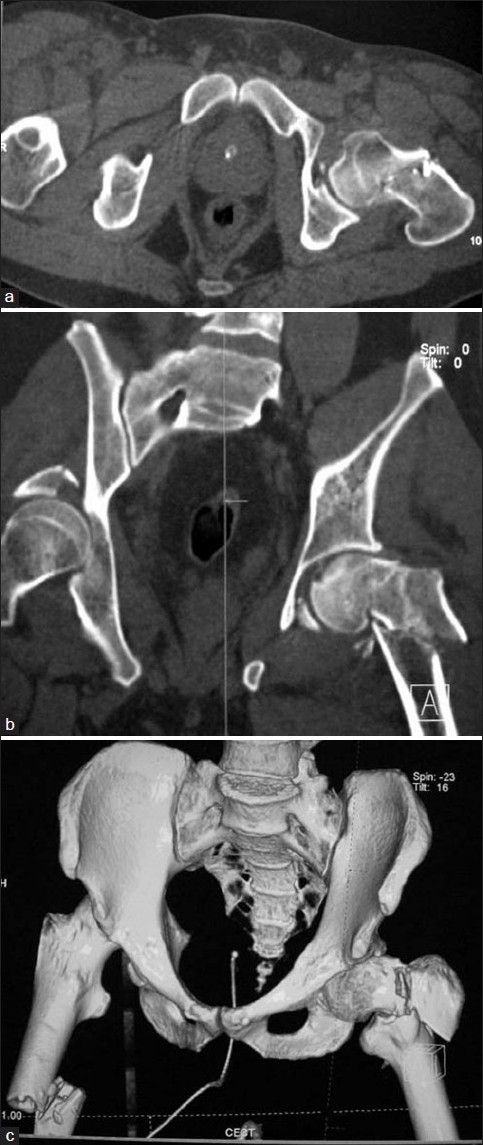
CT images of the pelvis including both hips. (a) A transverse section showing femoral neck fracture. (b) A section showing both femoral neck fracture and ipsilateral pertrochanteric fracture. (c) A 3D reconstruction image
